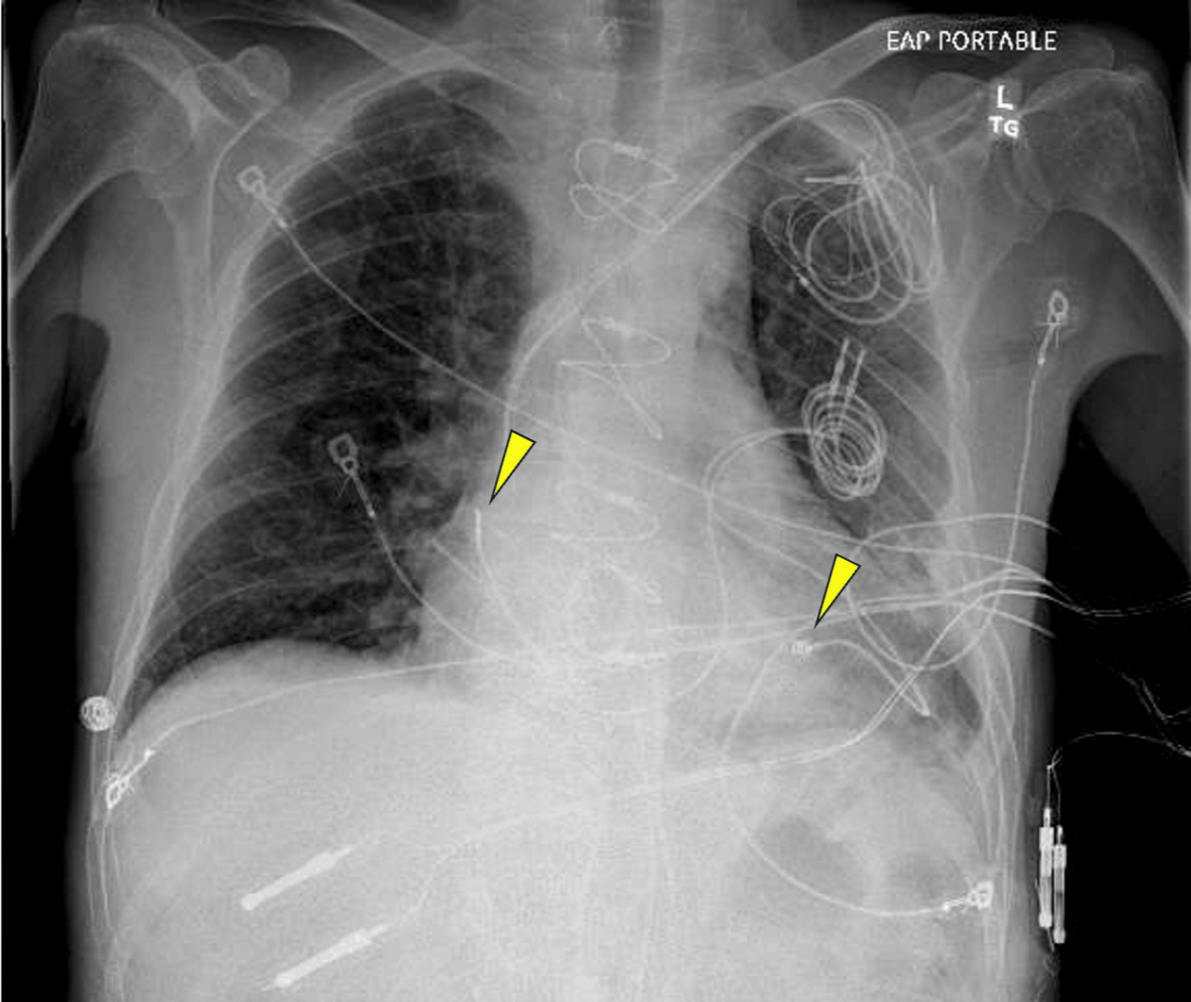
X-ray imaging of the endocardial lead placed on the epicardium of the right atrium (left arrow) and an epicardial lead placed on the surface of the right ventricle (right arrow).
